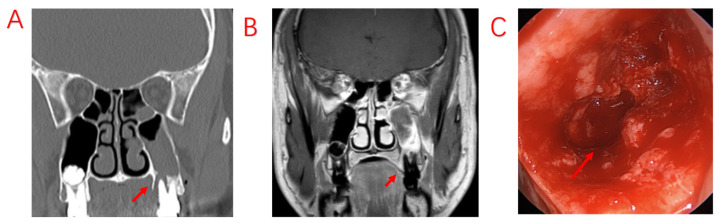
A patient with odontogenic sinusitis due to EPL with bone defects in the MSF. (A): Sinus CT shows resorption of alveolar bone on the palatal side of the left upper first molar, forming a narrow and deep periodontal pocket. (B): MRI shows diseased tissue in the periodontal pocket connected to swollen mucosa in the maxillary sinus. (C): Bone penetration of the palatal MSF in the extraction socket is observed during surgery. Red arrows indicate bony defects in the MSF.
